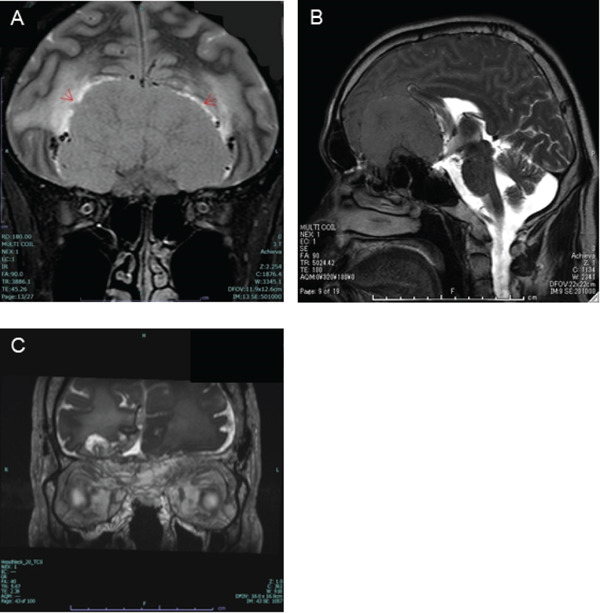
Findings on brain MRI. (A, B) At the initial presentation, a lobulated tumor measuring 7 × 6 × 4.5 cm (arrow) was found outside the brain parenchyma of the anterior base of the skull, severely compressing both frontal lobes and the corpus callosum. The tumor is isointense with the cerebral cortex on T1/T2WI and has no diffusion restriction. There are numerous flow voids at the edge and inside of the tumor. There is no noticeable compression of the optic chiasm. (C) At 3 months post‐operation, most tumor was removed, with no signs of increased intracranial pressure. A small amount of tumor remains. Abbreviations: MRI, magnetic resonance imaging; WI, weighted image.
